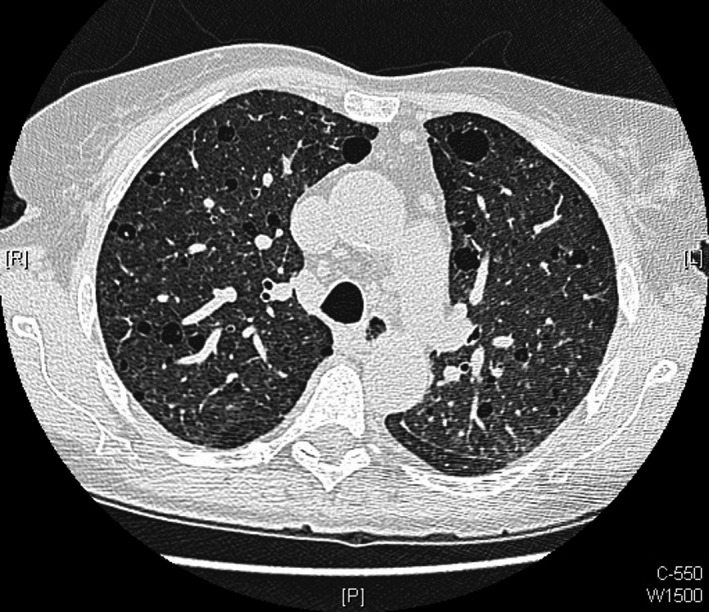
High‐resolution computed tomography (HRCT) chest showing progressive replacement of lung parenchyma with multiple cysts, and proliferation of abnormal smooth‐muscle cells, in a process termed lymphangioleiomyomatosis (LAM).
